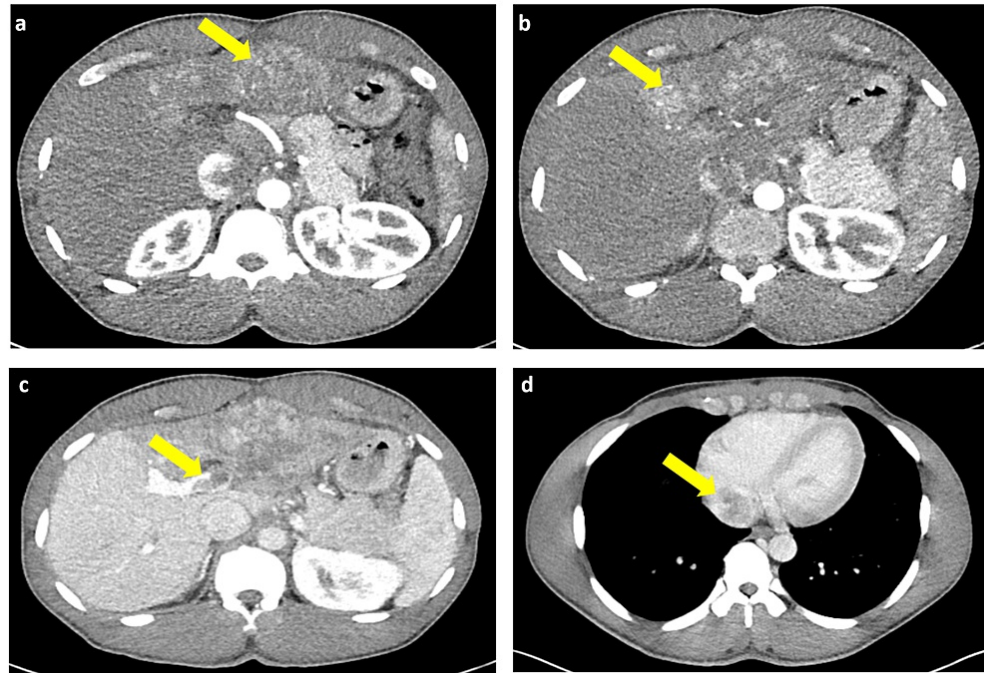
CT abdomen images CT: Computed Tomography a) CT abdomen arterial phase showed a heterogeneously enhancing partially exophytic hepatic lesion arising from the anterior left hepatic lobe, measuring 6 x 5.4cm; b) new heterogeneous enhancing lesion extends into segment 4, measuring 3cm; c) CT abdomen venous phase demonstrated increased enhancing tumor involving the left portal vein with complete obstruction; d) tumor extends into the main right portal vein, present in the left hepatic vein extending into inferior vena cava and right atrium.
